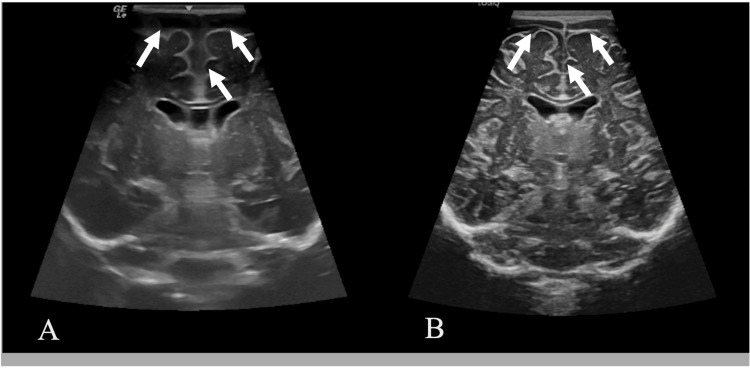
Cranial Ultrasounds at Admission and Two Months After Initiation of Treatment At admission (A), bifrontal and anterior hemispheric extra-axial cerebrospinal fluid spaces were prominent (arrows). On follow-up two months after treatment initiation (B), there was a marked reduction in the prominence of these spaces (arrows), consistent with therapeutic response.
